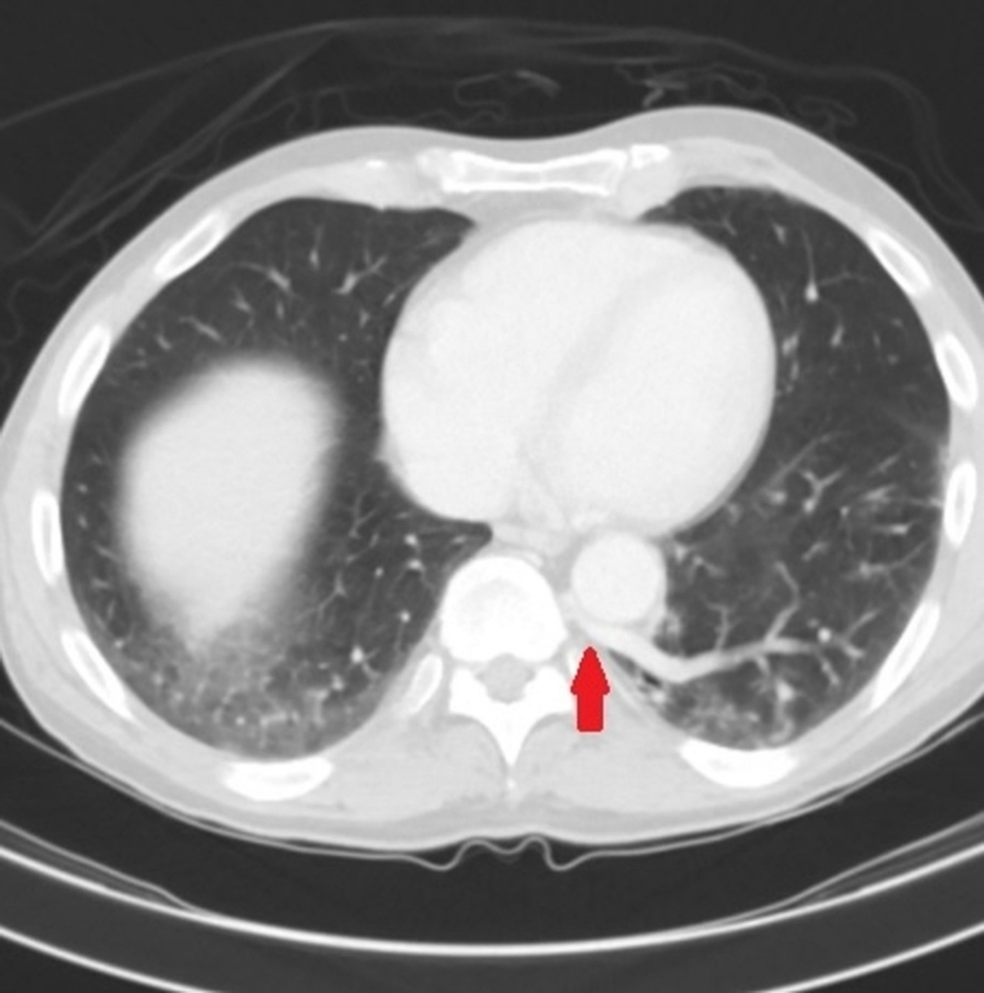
Axial CT scan of the chest with contrast demonstrating anomalous pulmonary vein arising from intralobar sequestration
